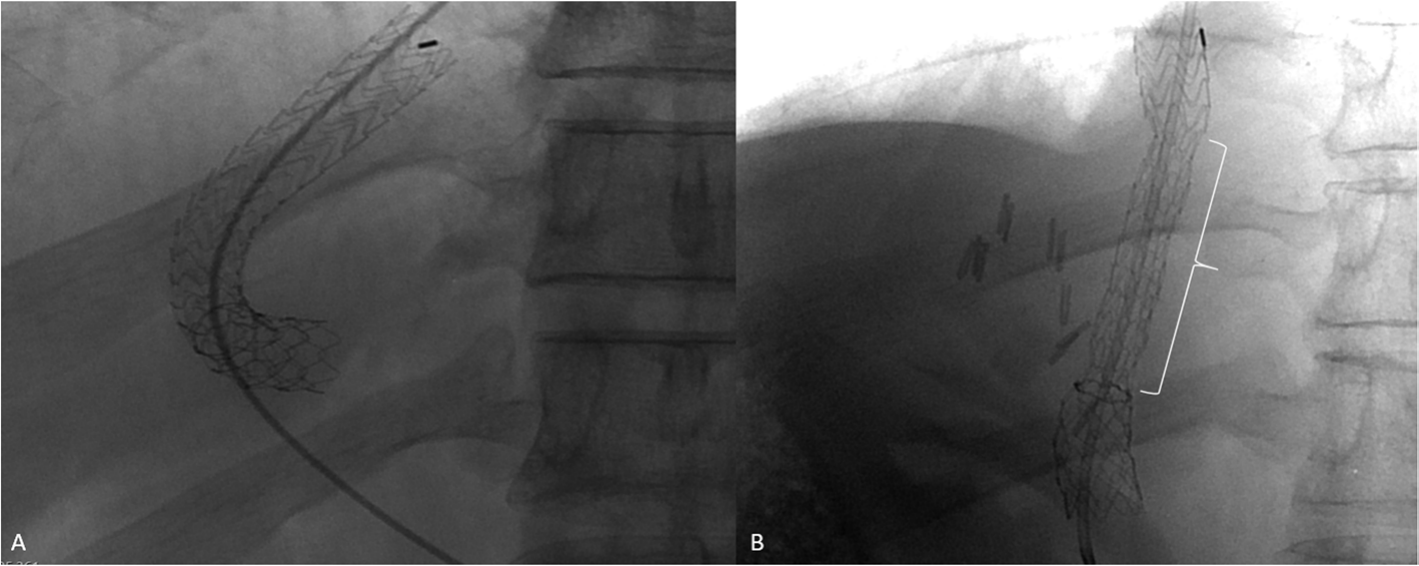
Radiographic images after deployment of the Legacy stent-graft (A) and the VCX stent-graft (B). Notice the diameter is the same (10 mm) along the Legacy stent-graft but constrained (8 mm) in the VCX stent-graft (highlighted), where an extra-sleeve prevents further passive expansion to the nominal (10 mm) diameter
